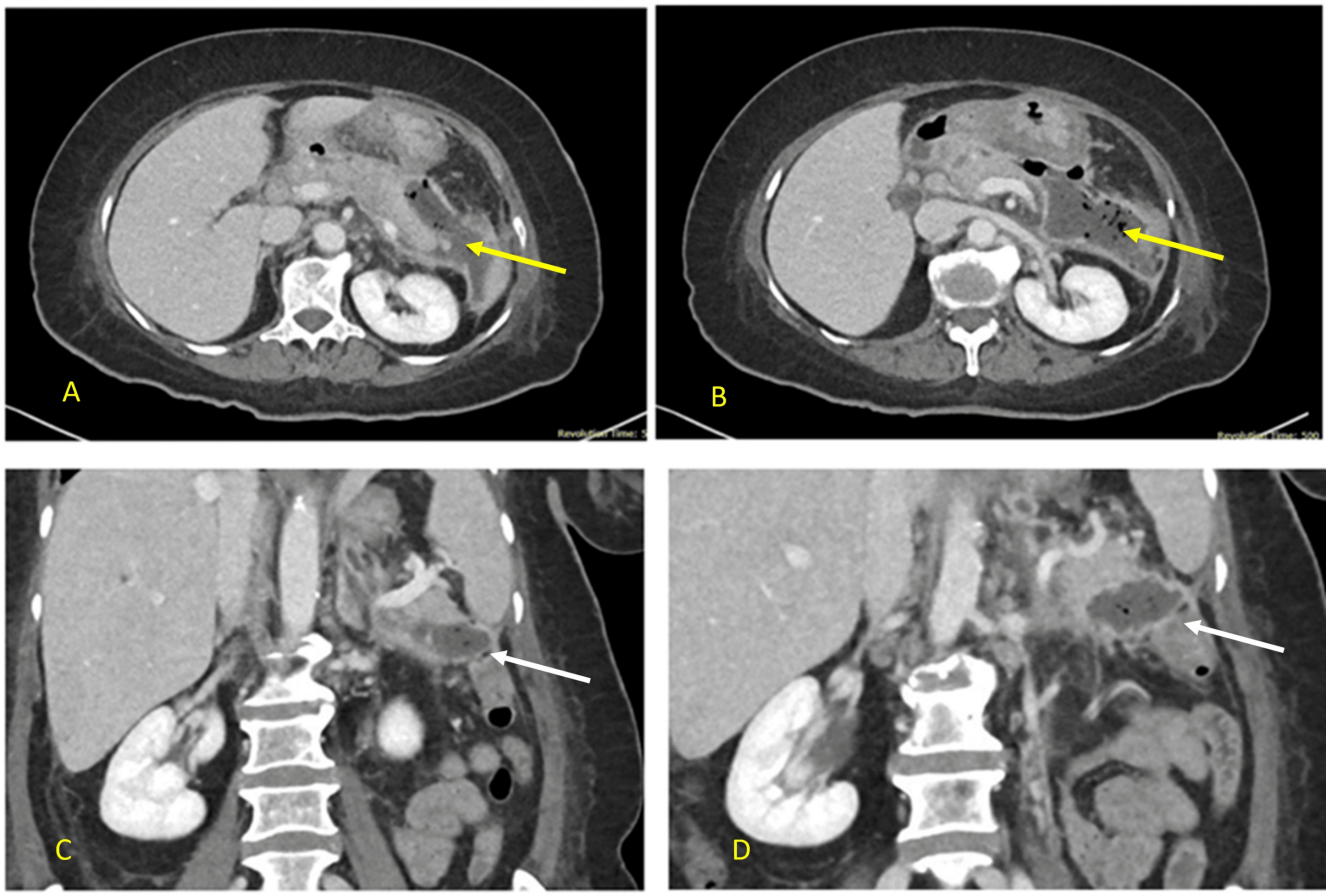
CT scan of the abdomen showing a pancreatco-colonic fistula A and B: The axial section shows a persistent collection with multiple air pockets noted in the tail of the pancreas, with its limbs extending towards the neck (yellow arrow). C and D: a coronal view showing a peripancreatic collection communicating with the splenic flexure, indicative of a fistula (white arrow).
